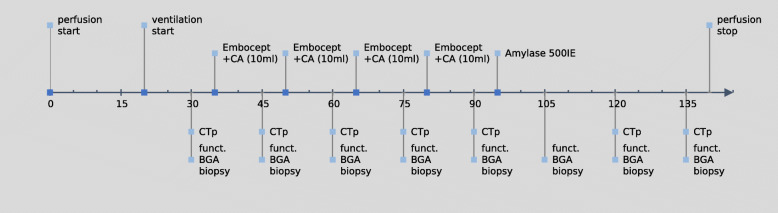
Timeline of the experiment. *BGA* Blood gas analysis, *CA* Contrast agent, *CTPI* Computed tomography perfusion imaging, *Funct.* Functional assessment. *x* axis values given in min
